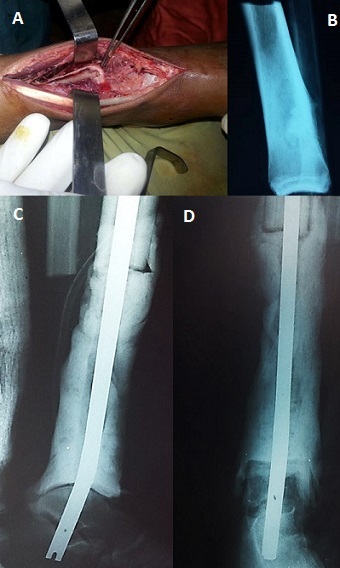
(A) per operative aspect; (B) a wide resection of tibia and fibula along 12 cm from the tibio-talar joint performed with a tumour free margin of 3 cm; (C,D) post-operative plain radiographs
